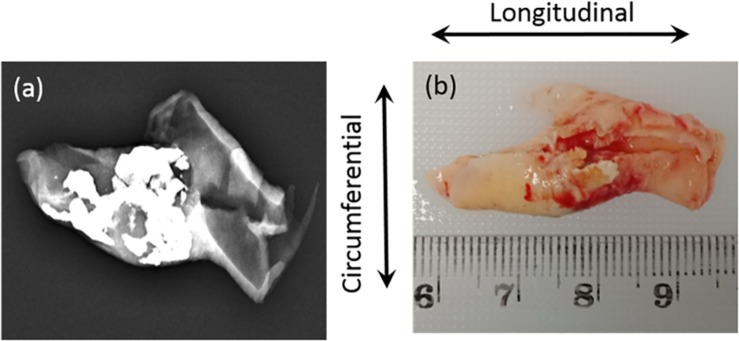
(a) Low energy X-ray used to identify the calcium content; (b) Sample collected from hospital with cutting direction (circumferential).
